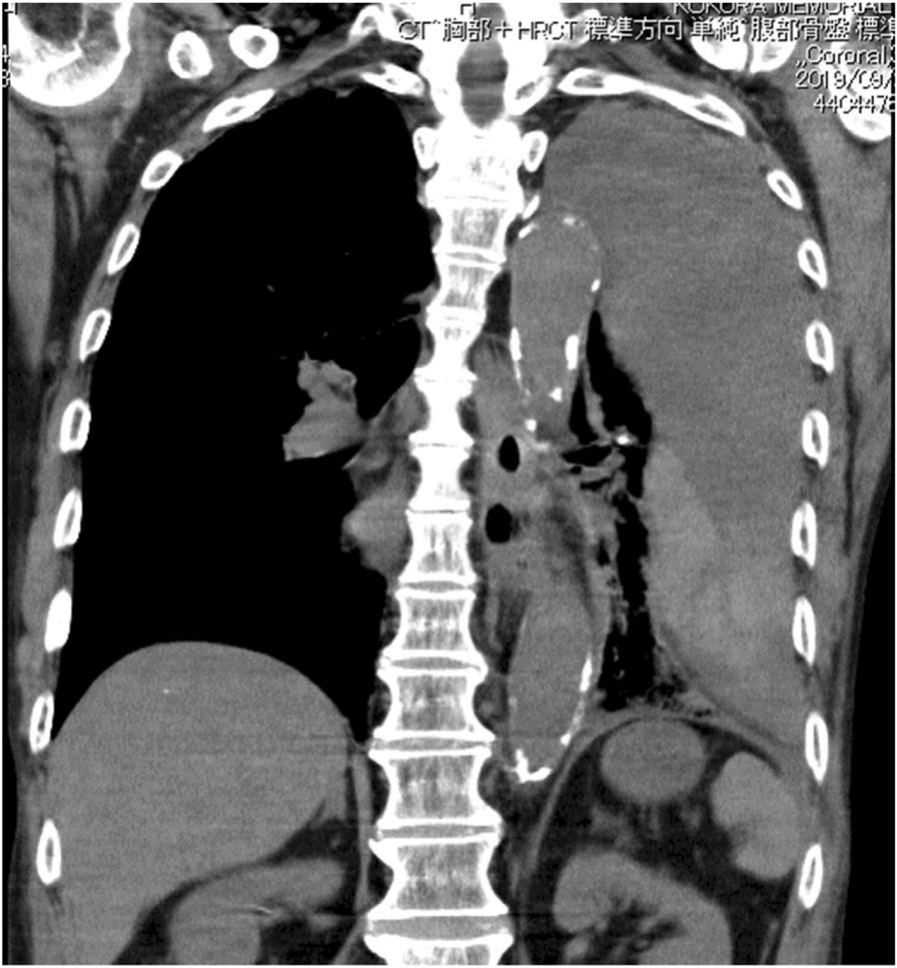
Chest computed tomography (CT) scan showing a hematoma in tt extrapleural space
